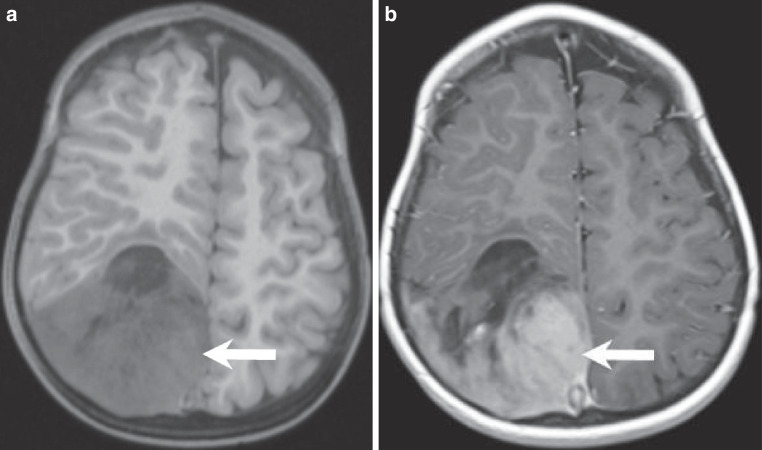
Axial pre-contrast T1-weighted MR images (a) revealed a hypointense signal of the solid tumour components (arrow). Axial T1 weighted images after gadolinium administration (b), showed marked contrast enhancement of the solid tumour portion
